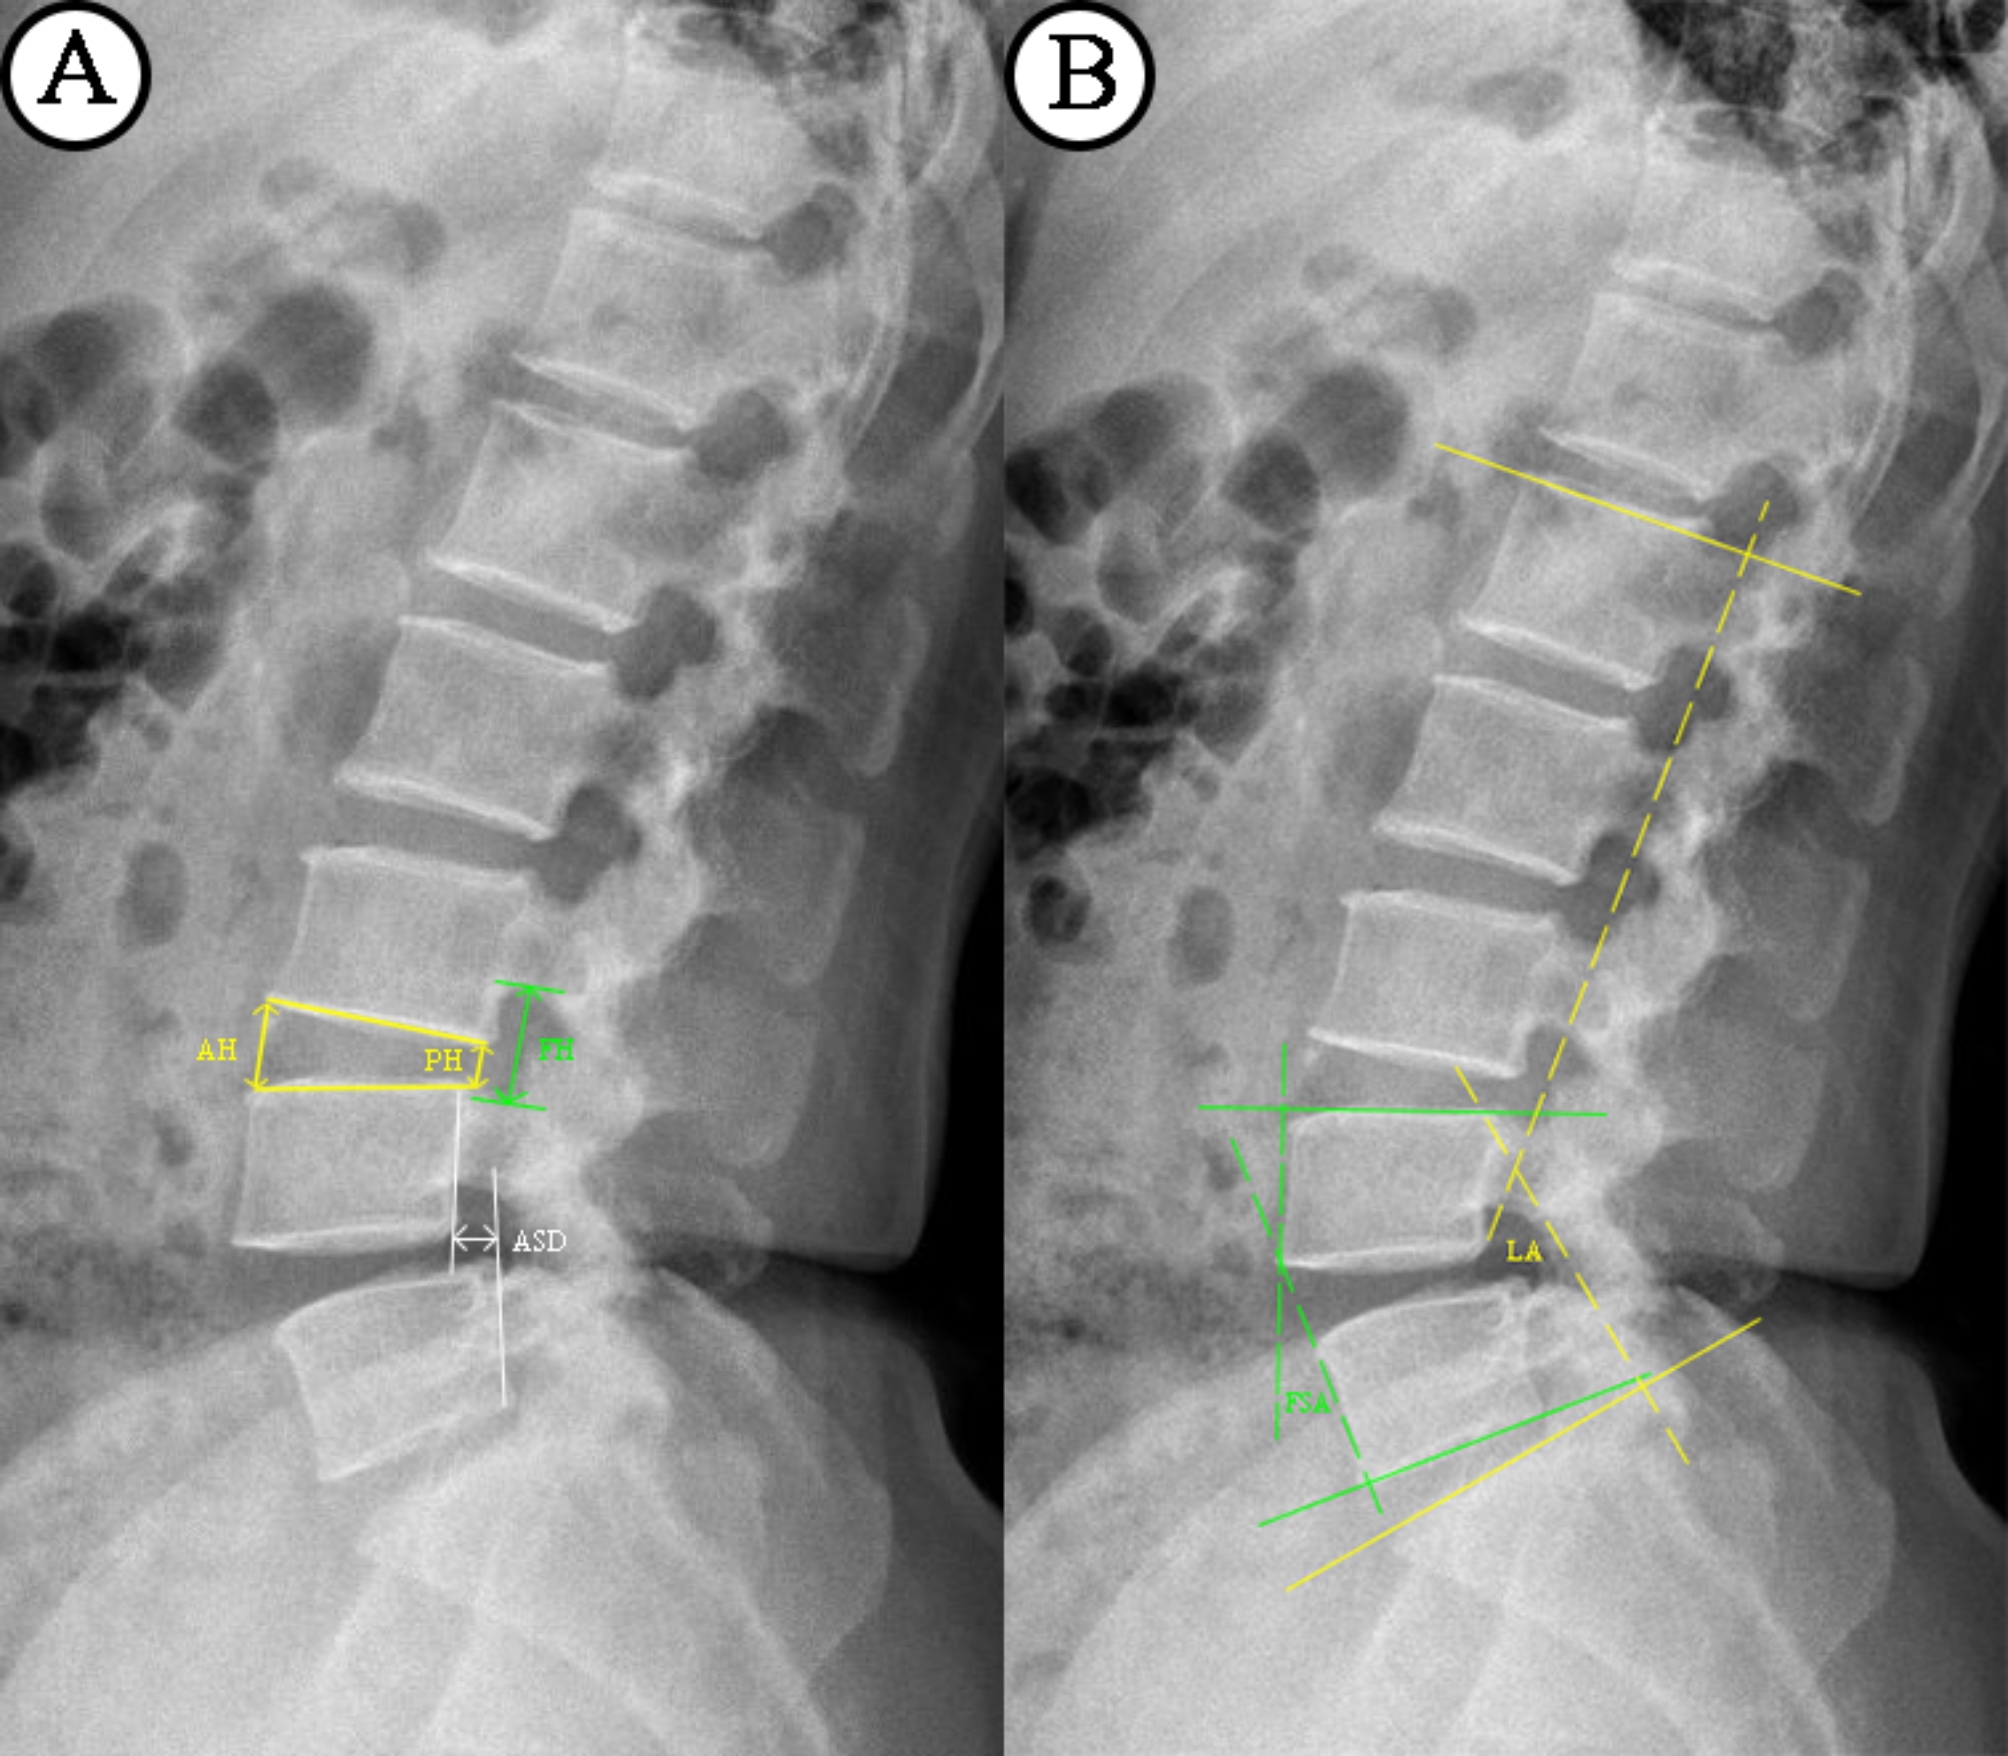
Length parameters measured on lateral X-rays (A). Disc height= (AH + PH)/2; foraminal height = FH; Anterior spondylolisthesis distance = ASD. Angle parameters measured on lateral X-rays (B). Segmental lordotic angle = FSA; lumbar lordotic angle = LA.
